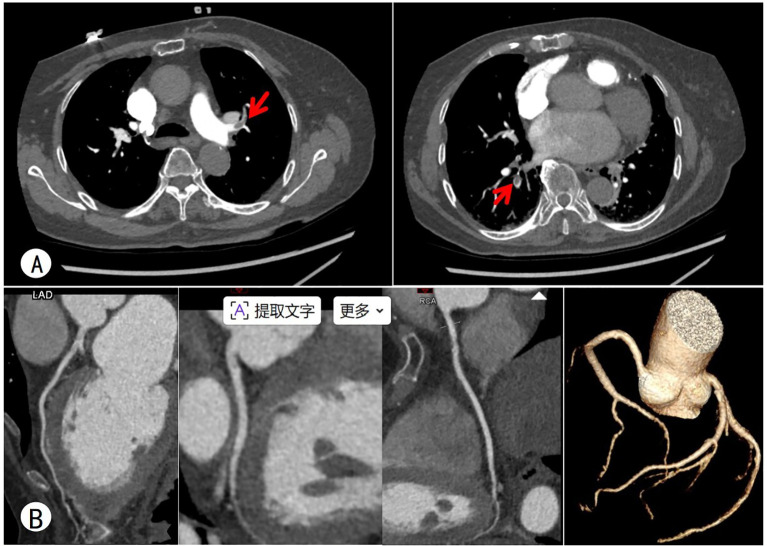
(A) Pulmonary artery CTA: Embolism in partial branches of bilateral pulmonary arteries. (B) Coronary CTA: approximately 30% stenosis in the LAD and approximately 30% stenosis in the RCA.
